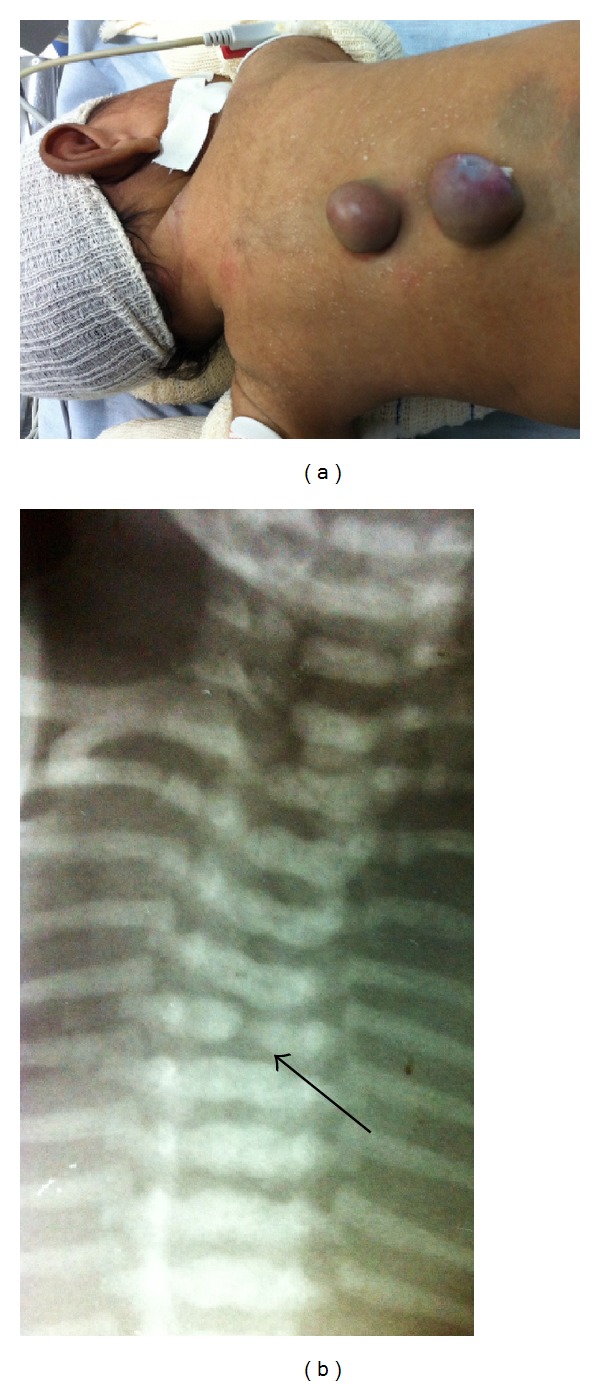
(a) A newborn with multiple thoracic myelomeningocele. (b) Plain radiograph of the chest, showing a reduction and a fissure in the body of the fifith thoracic vertebrae.
